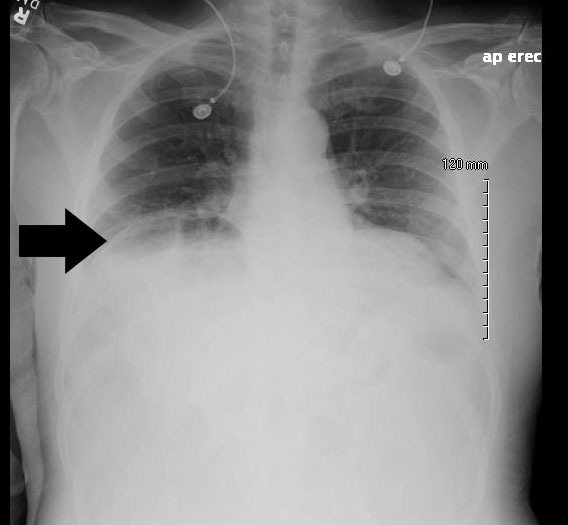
Upright chest radiograph demonstrating Chilaiditi sign (pseudopneumoperitoneum) mimicking apparent free intraperitoneal air under the right hemidiaphragm.
